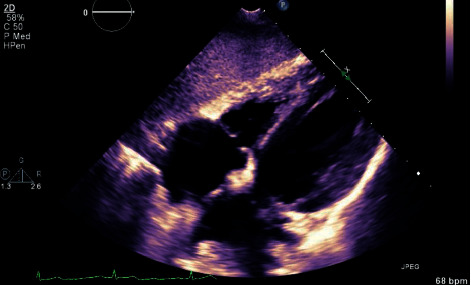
Transthoracic echocardiogram (TTE) after antifungal treatment with resolution of the large fungal mass.
